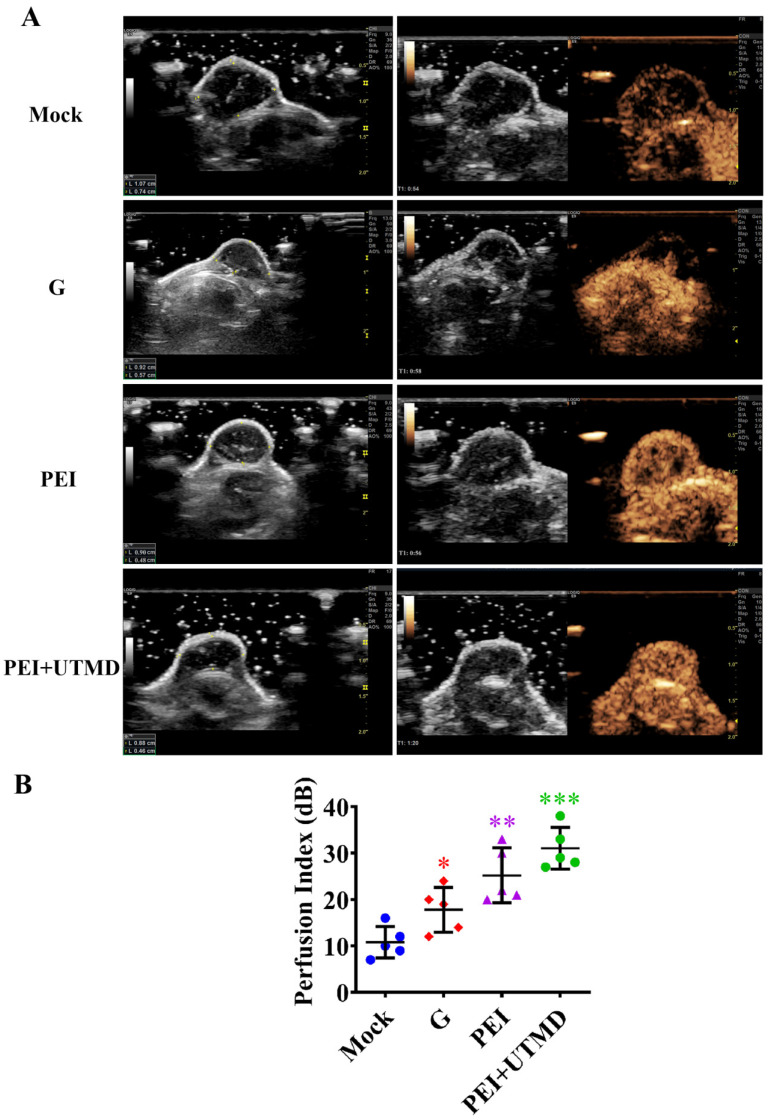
Polyethyleneimine (PEI) nanohydrogel treatment increases pancreatic ductal adenocarcinoma (PDAC) tumor blood perfusion, which is further enhanced by adding ultrasound targeted microbubble destruction (UTMD). (A) The blood perfusion of microvessels in tumors can be observed in real time using contrast-enhanced ultrasound (CEUS). (B) The quantitative analysis of blood perfusion in the different groups.
